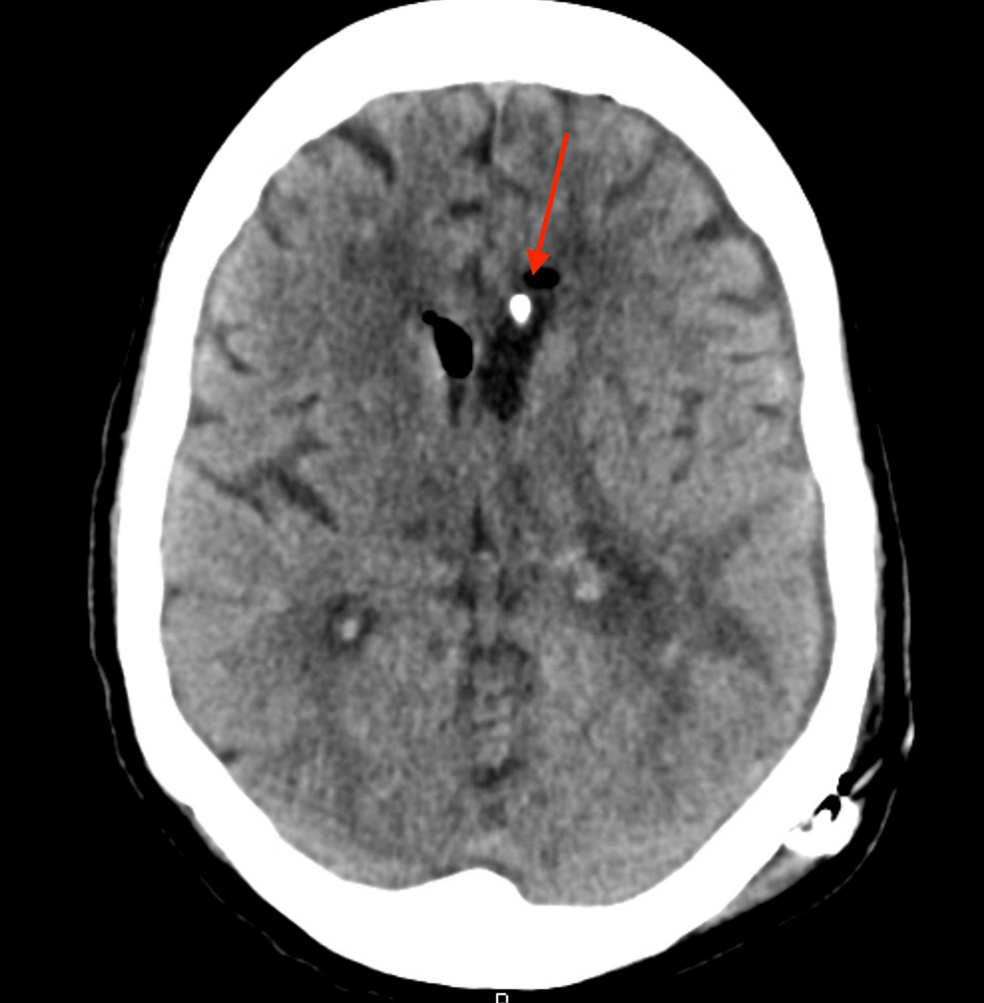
Head CT following ventriculoperitoneal shunt placement Arrow indicates the tip of the shunt catheter
